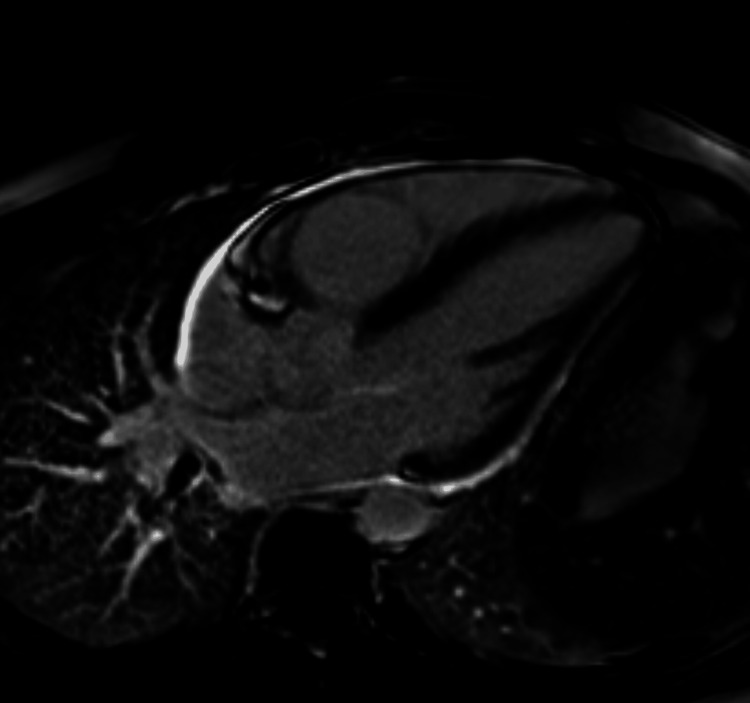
MRI cardiology late gadolinium 3 chambers image. Acquired 10 minutes after administration of IV Gadovist using an inversion time of 300 ms, revealing the normal appearance of LV and RV and no CMR features of myocarditis.
